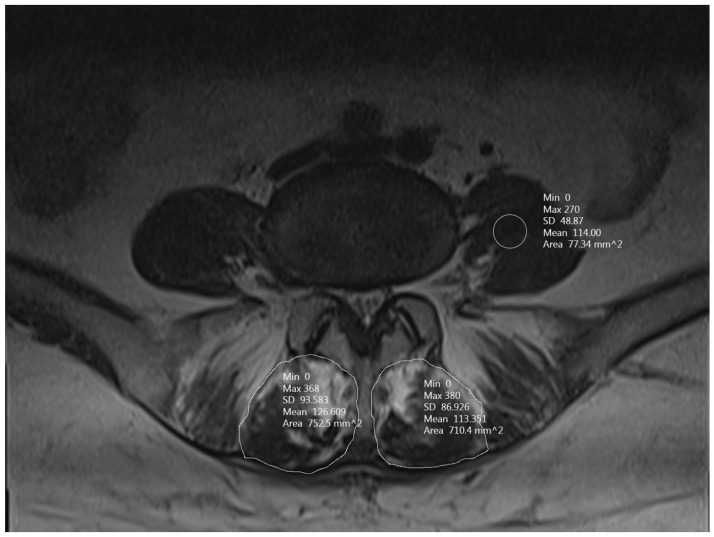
Multifidus muscles (MFMs) on MRI after surgery.
